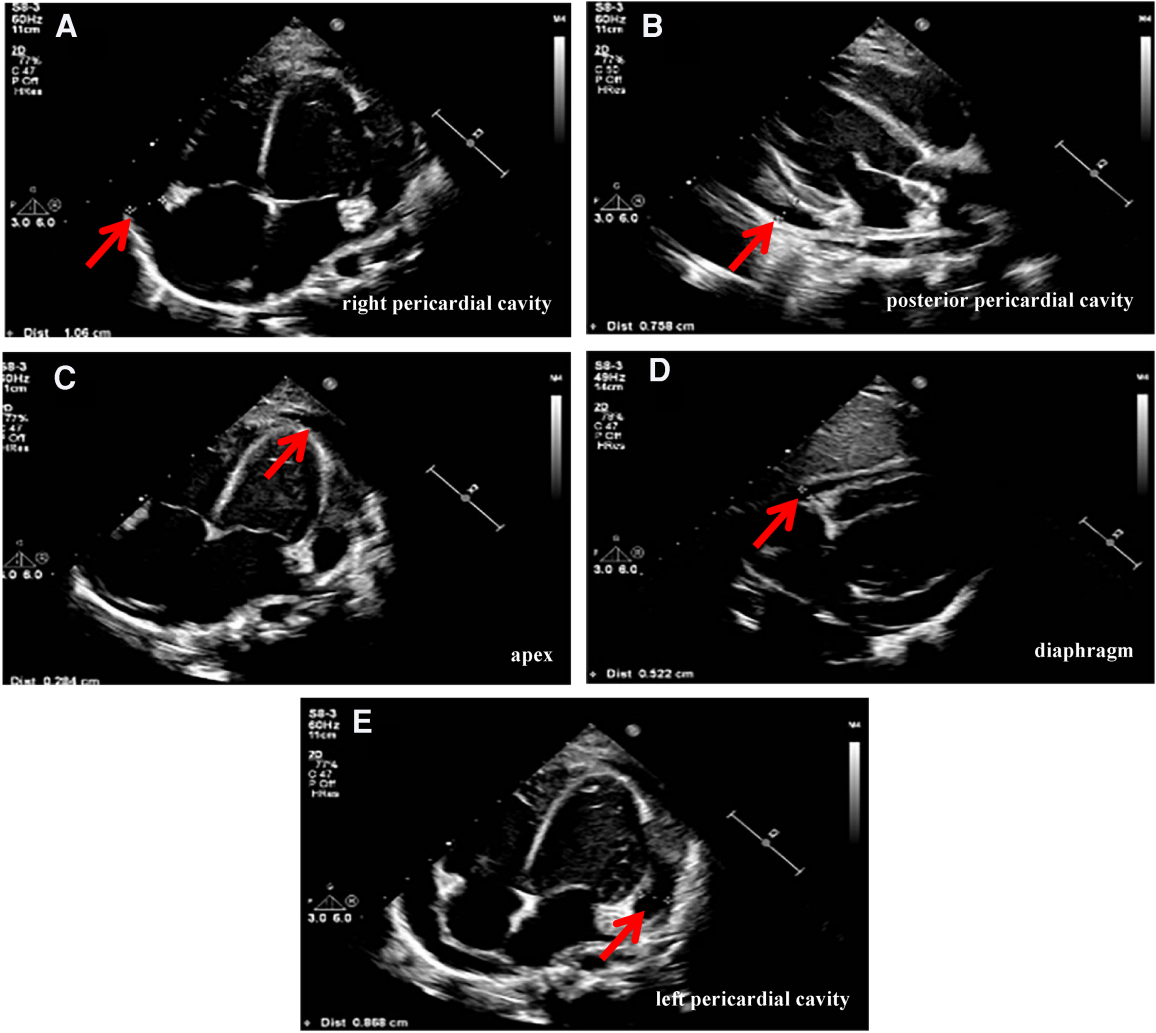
Echocardiography (on admission) showed pericardial effusion: (A) posterior pericardial cavity of 7.6 mm. (B) Left pericardial cavity of 8.7 mm. (C) Right pericardial cavity of 10.6 mm. (D) Apex of 2.8 mm. (E) Diaphragm of 5.2 mm.
